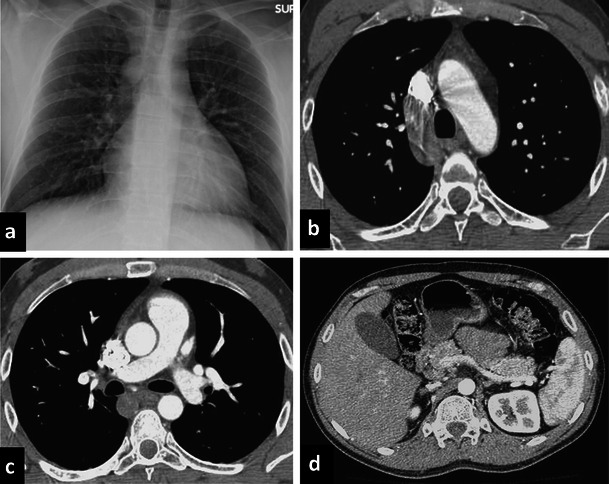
Azygos continuation. Chest X-ray shows enlargement of the azygos vein (a) on the right superior mediastinum. Axial enhanced CT shows an enlargement of the azygos vein (b–c) and absence of the inferior vena cava with azygos continuation (d)
